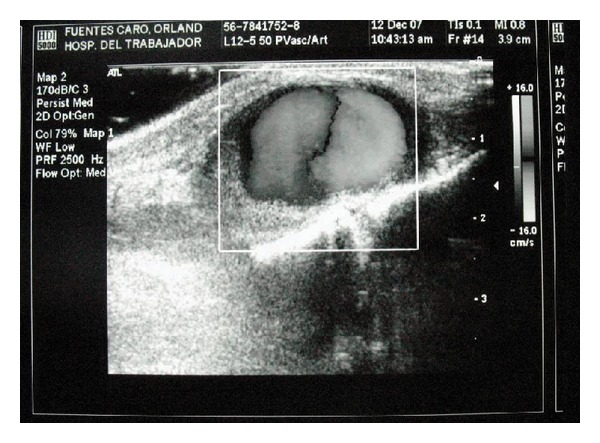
Color Doppler ultrasound demonstrating an hypoechoic 2.64 × 1.53 cms lesion.
